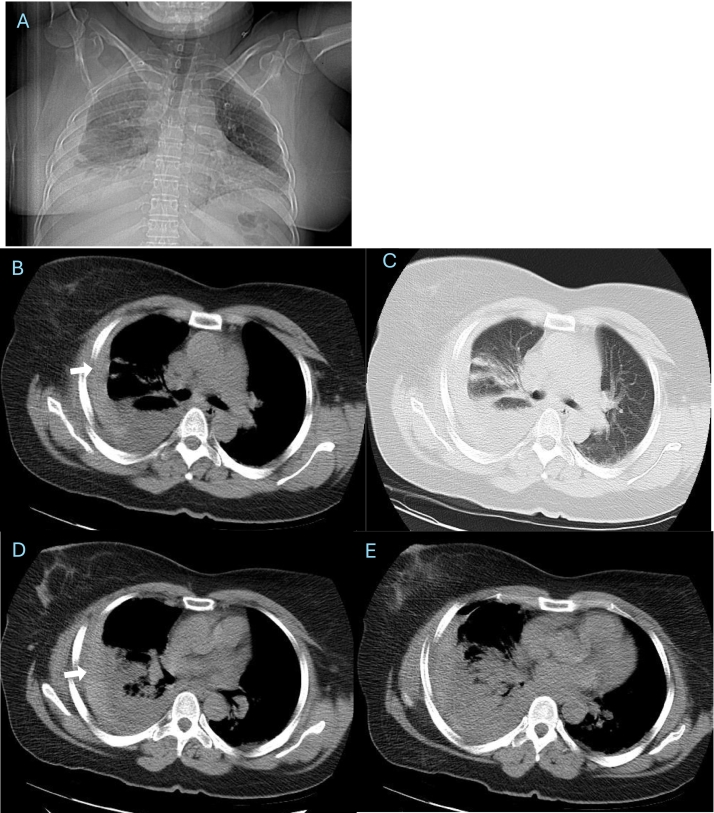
Scout and axial CT images in the supine position acquired 6 h after biopsy showing substantial enlargement of the right pleural effusion (white arrow) with compressive atelectasis of the lower lobe. The persistent high-density fluid supports ongoing hemothorax.
